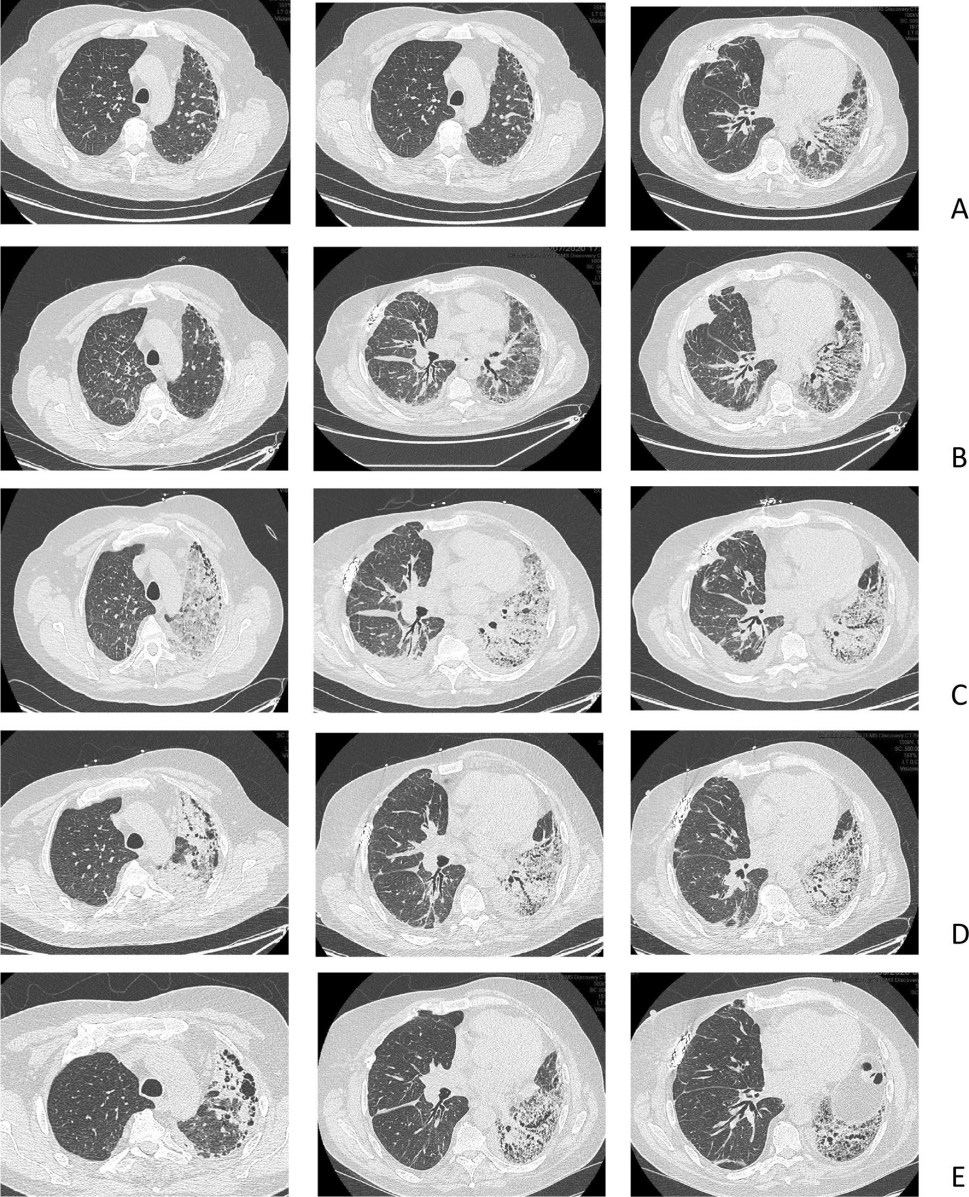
CT of the thorax in patient 1, a 65-year-old man, at various times after right single lung transplantation (SLT) for idiopathic pulmonary fibrosis (IPF). A Day 7 after hospital admission (day 1) for management of CMV infection in a patient with CMV mismatch. B Day 14, 1 day before the patient developed acute respiratory failure (ARF) requiring a transfer to the intensive care unit (ICU) on day 16. C Day 19, while in the ICU for ARF. D Day 27, 1 day before discharge from the ICU. E Day 63, well after the ARF episode.
